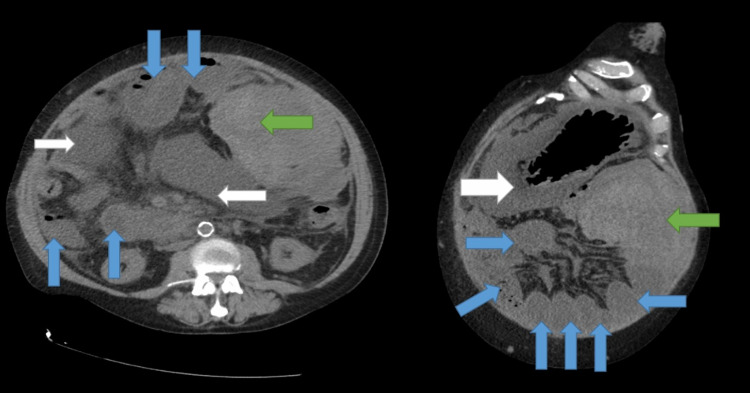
CT abdomen and pelvis one week after initial discharge Axial view (left) and coronal view (right) demonstrate a large peritoneal hematoma (green arrows), dilated small bowel loops (blue arrows), and large ascites (white arrows) CT: computed tomography
